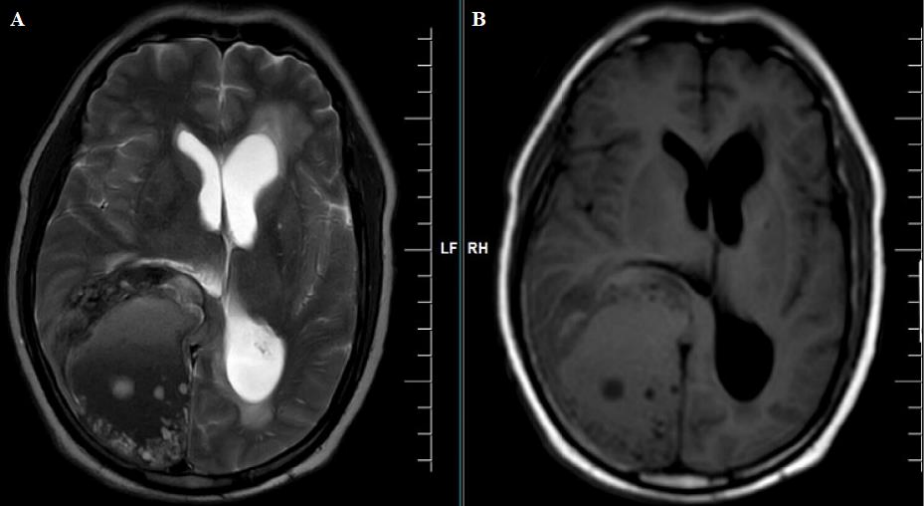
A,B) MRI of the brain axial section, lesion in right occipital lobe appearing hypointense on T1-weighted image (T1WI) and hyperintense on T2-weighted image (T2WI) with midline shift to left
